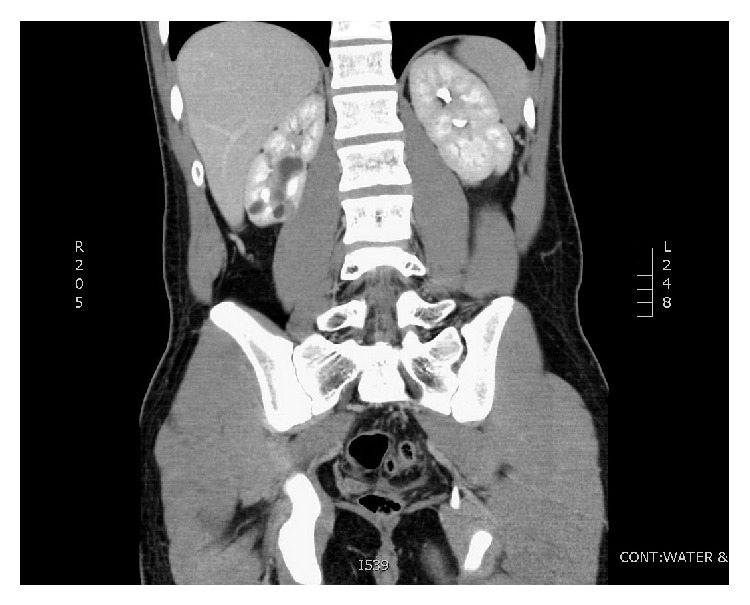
CT scan with contrast showing dilated calyces and associated cortical thinning in the lower pole of the right kidney. The left kidney appears normal.
